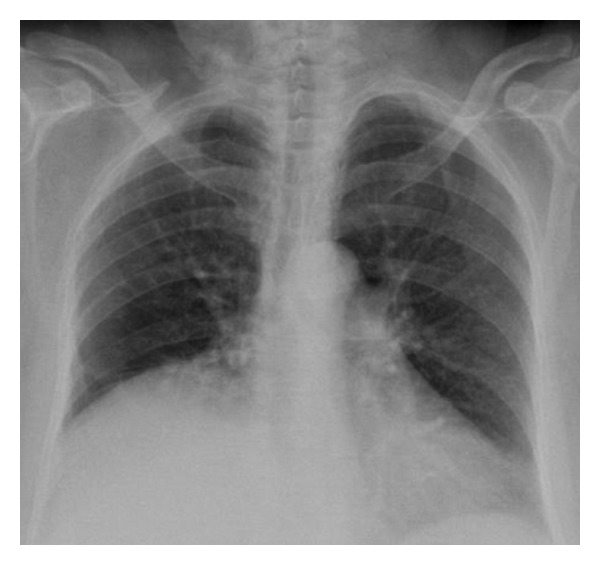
On the PA chest radiography, the volume of the right hemithorax was decreased and the hemidiaphragm elevated. The right pulmonary hilus was small, and hyperlucency and decrease of peripheral vascular shadowing of the right lung were detected.
